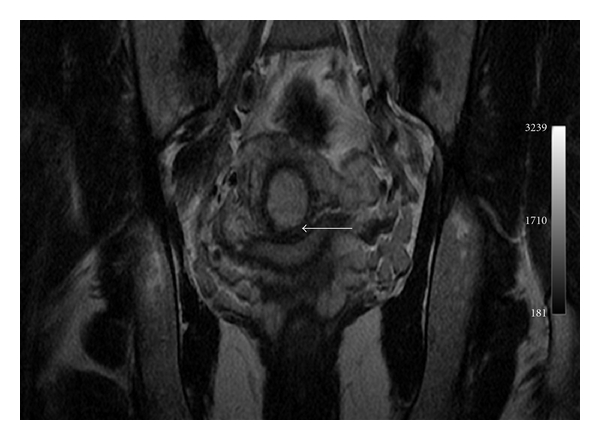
Magnetic resonance imaging gestational sac surrounded by myometrium (arrow head), absence of myometrium between the gestational sac and the bladder (arrow).
